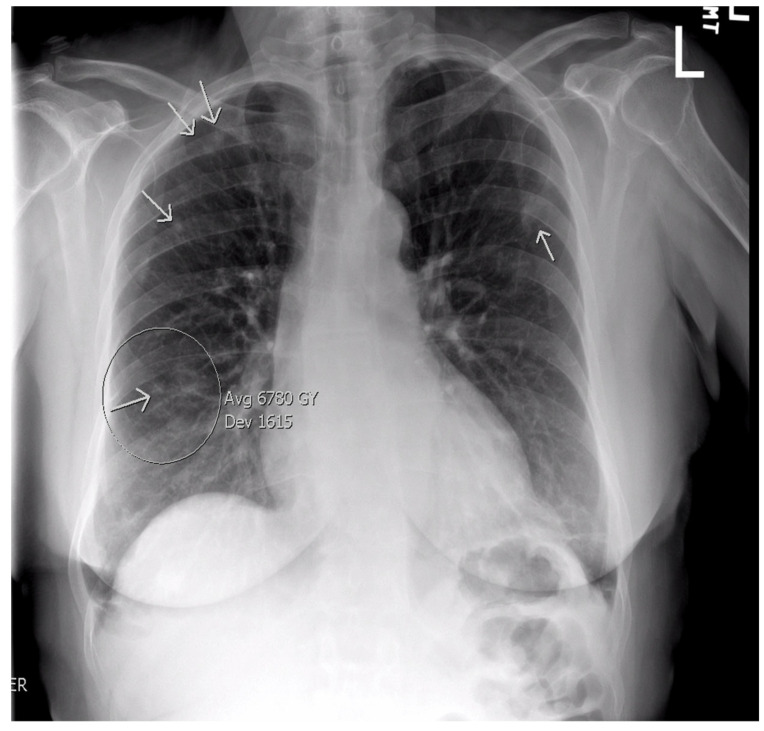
Chest X-ray revealing four nodules in the right lung and one in the left lung (white arrows).
